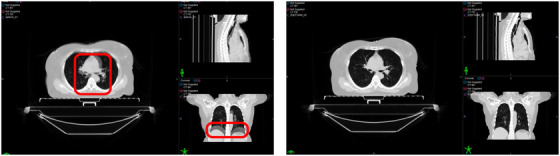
Left: Image co‐registration between FB and BH CT scan using deformable algorithm of velocity AI (red boxes highlight inferiority in image co‐registration using deformable algorithm). Right: Image co‐registration between FB and BH CT scan using extended deformable multi‐pass algorithm of velocity AI.
